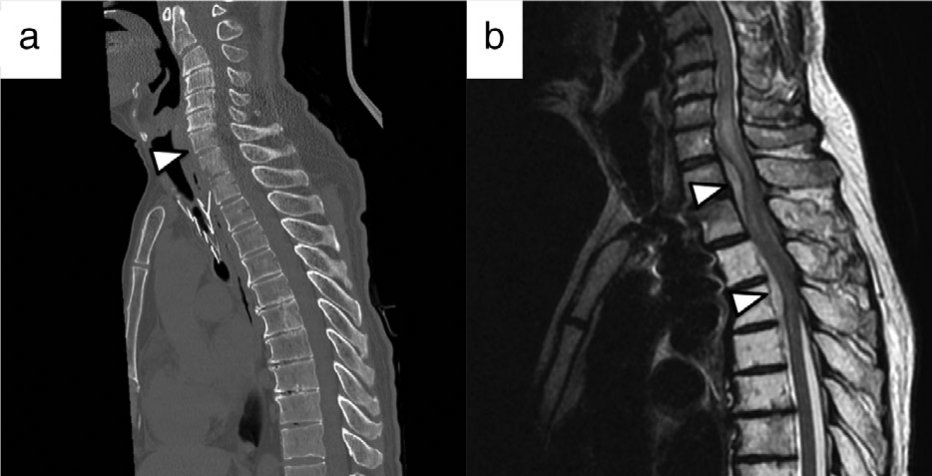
Imaging findings on admission. (a) Computed tomography (CT) scan revealed an osteolytic lesion at the T1–T2 disc level, adjacent to the fractured stent. (b) T2‐weighted magnetic resonance imaging demonstrated high signal intensity in the disc space, with an epidural abscess compressing the spinal cord.
